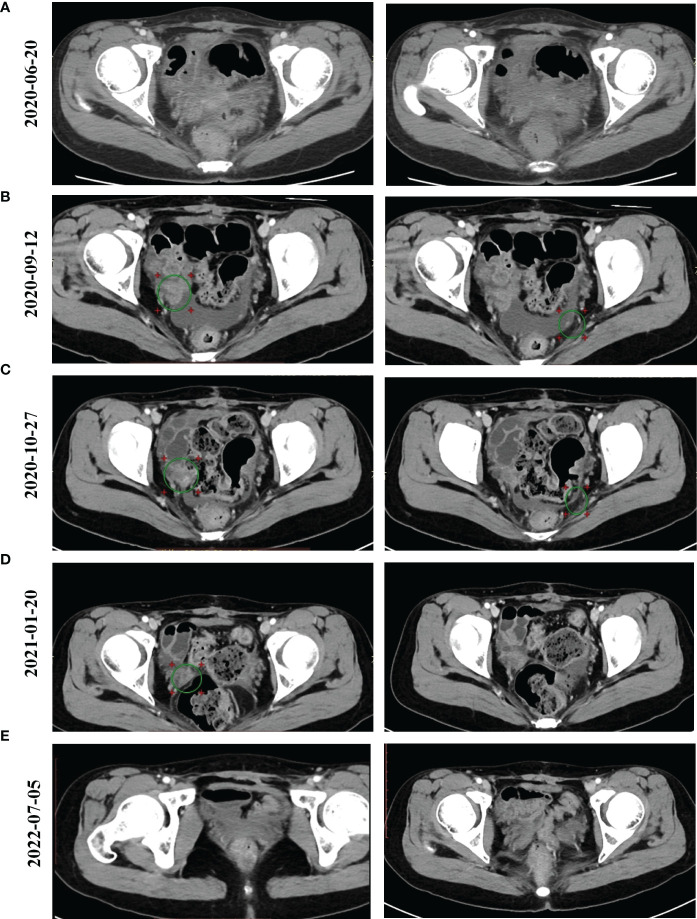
Computed Tomography (CT) images for our patient over the course of treatment. (A) The results of abdominal enhanced CT scan for the patient following two cycles of trastuzumab/pertuzumab/capecitabine therapy after the patient underwent radical surgery on 20 June 2020. (B) The results of abdominal CT scan for the patient following six cycles of trastuzumab/pertuzumab/capecitabine therapy on 12 September 2020. (C) The results of abdominal CT scan for the patient following two cycles of pyrotinib/bevacizumab/capecitabine therapy on 27 October 2020. (D) The results of a PET-CT scan for the patient following six cycles of pyrotinib/bevacizumab/capecitabine therapy on 20 January 2021. (E) Positron Emission Tomography-CT (PET-CT) scan showed our patient’s condition on 5 July 2022.
